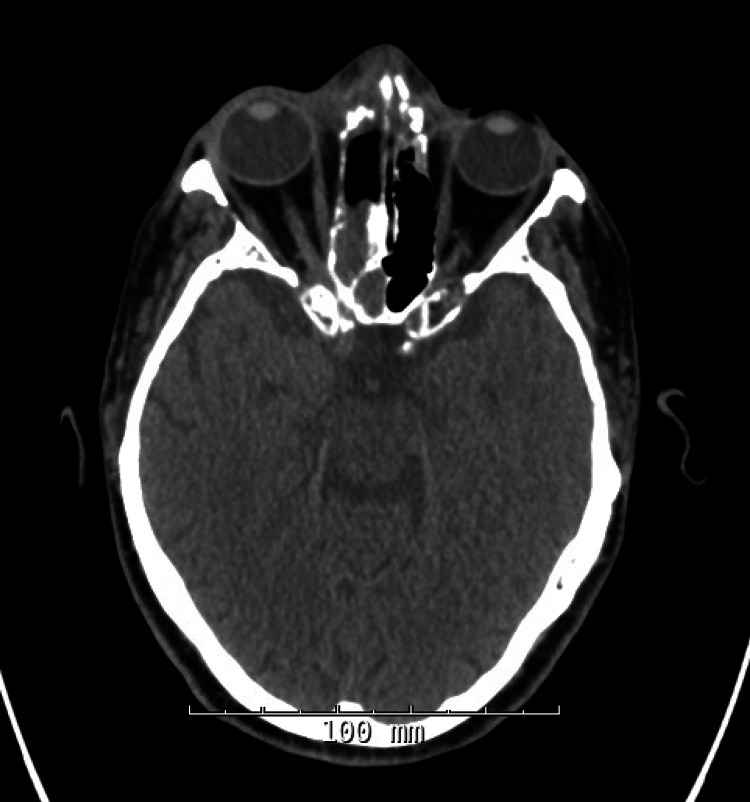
CT Scan With Multifocal Sinusitis
